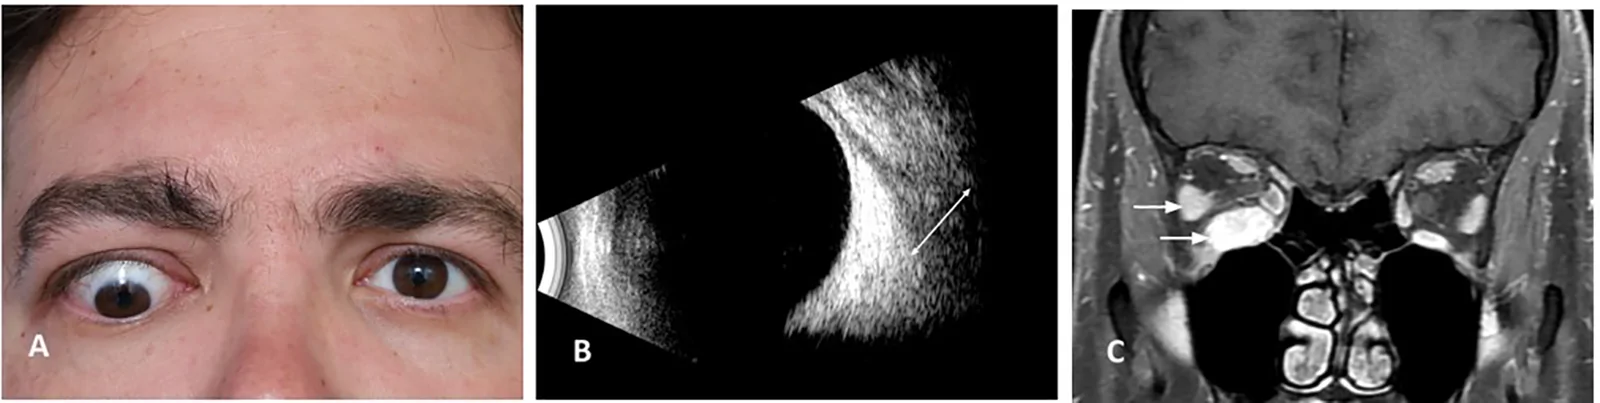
Idiopathic Orbital Myositis. (A) External photography of a 36-year-old patient with recurrent inflammation of the right inferior rectus. (B) Longitudinal B-scan shows a low reflective enlargement of the right inferior rectus (double arrow). (C) Coronal T1-weighted MRI shows an enlargement of the lateral and inferior recti muscles (arrows).
